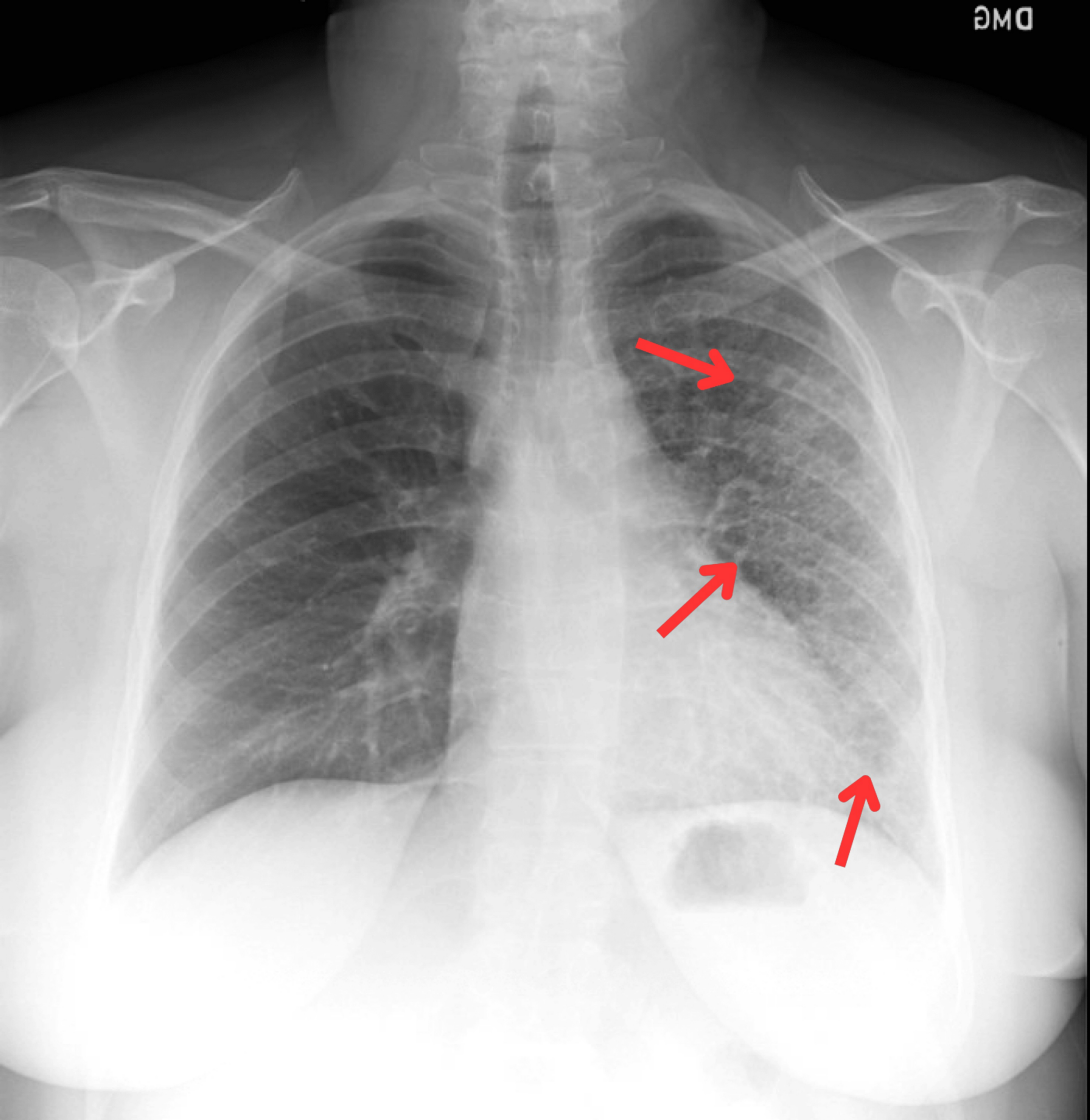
Chest radiograph (posteroanterior view) showing subtle hazy opacities and reticulonodular markings with volume loss predominantly in the upper and mid zones of the left lung. These findings were initially interpreted as consistent with atypical infection or inflammatory bronchiolitis. The red arrows represent increased reticular markings.
